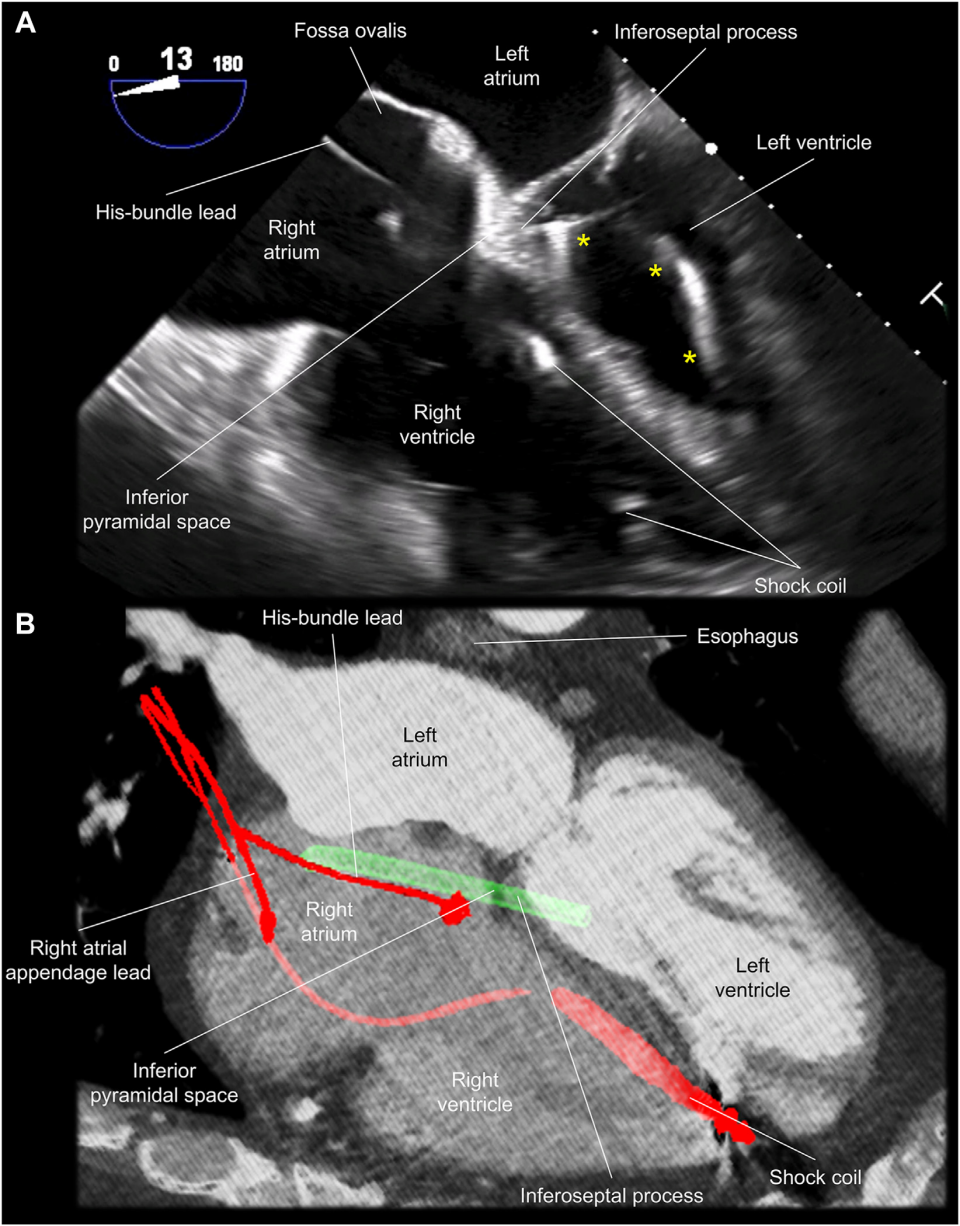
Transesophageal echocardiography. A: Transesophageal echocardiography shows the catheter (*asterisks*) placed within the left ventricle after passing through the inferior pyramidal space and inferoseptal process. B: replicates the sectional plane of the transesophageal echocardiography (A) with a virtual sheath (*green*).
